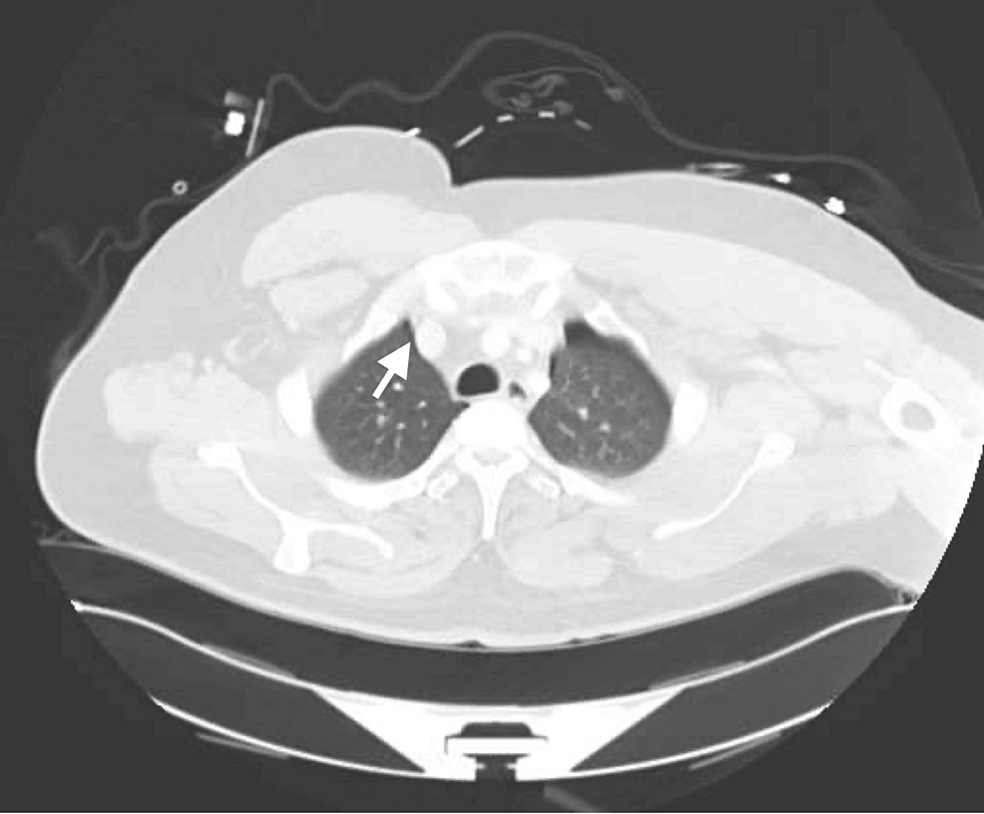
CT scan of the abdomen shows a left retroperitoneal hemorrhage.
